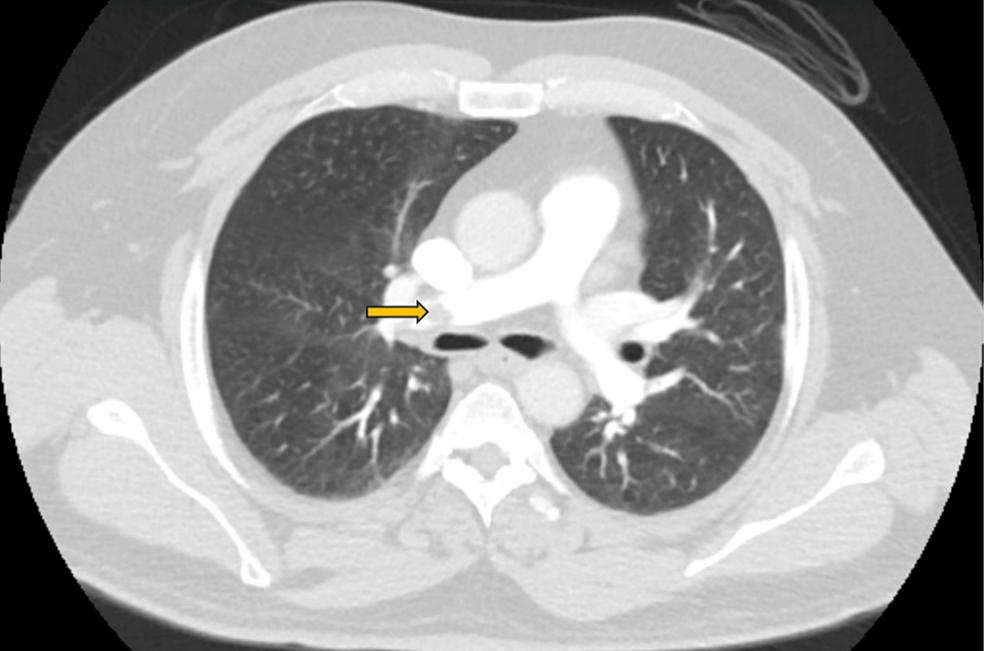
CT angiogram of the chest at the level of main pulmonary artery branches shows emboli (arrow) (2021)
